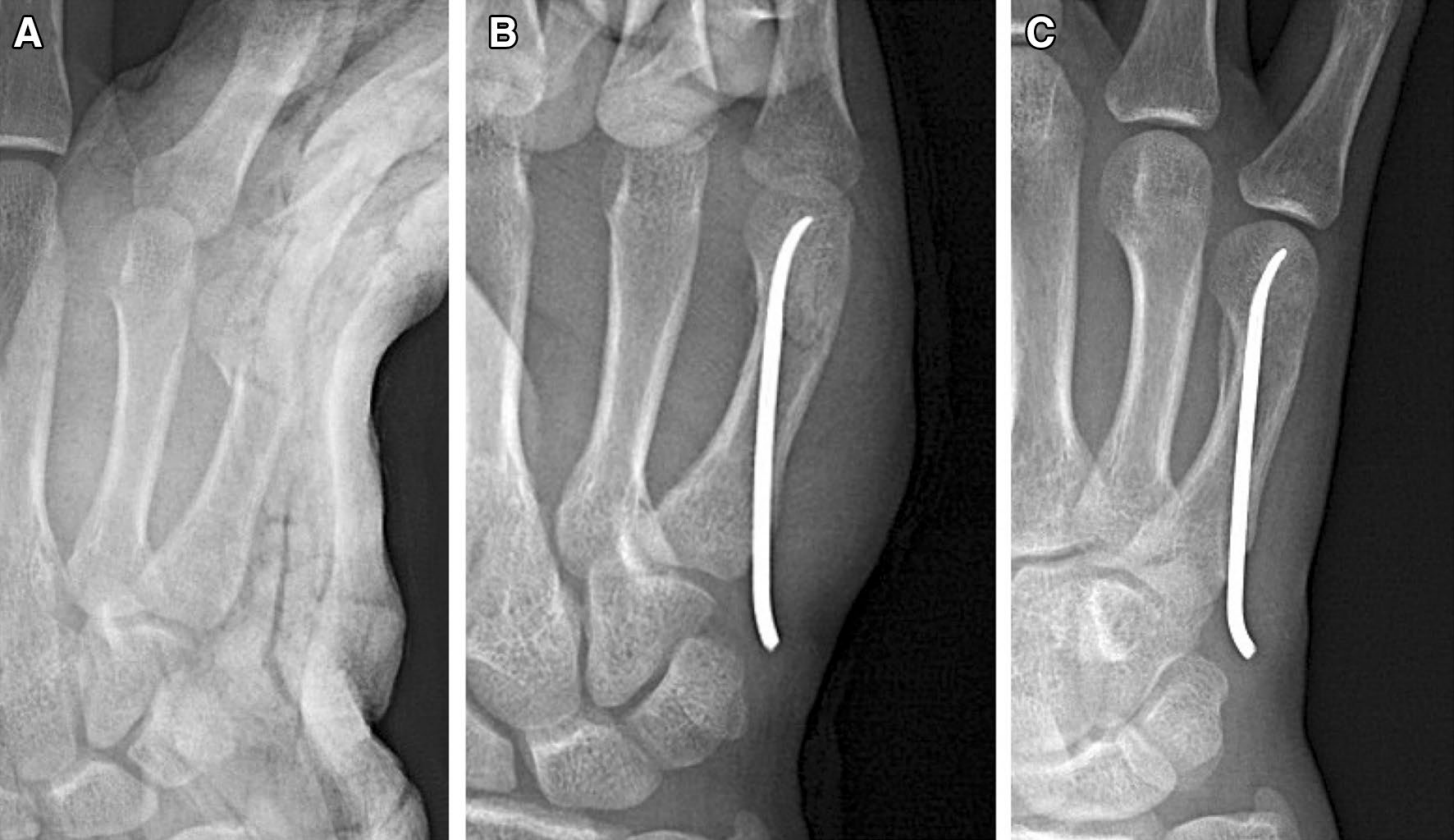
Clinical case of an FMNF treated by single AEIMN. Preoperative oblique view radiograph (A). Postoperative radiographs showing a good reduction of the fracture (B). Radiograph at 8 weeks after the operation shows union of the fracture (C).
